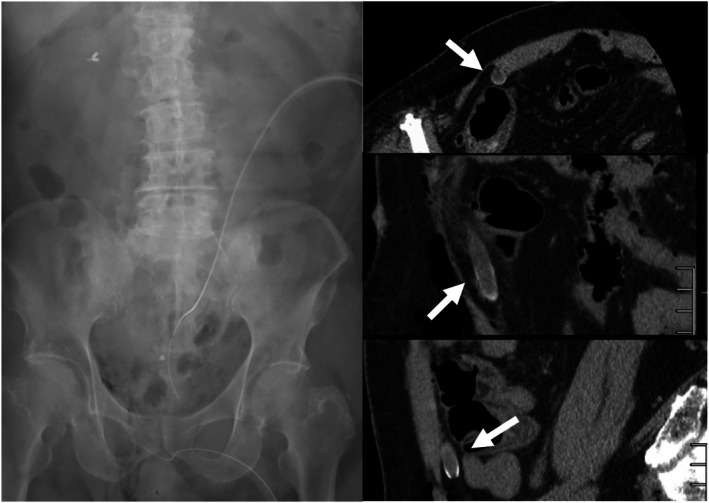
Postoperative imaging for detection of the retained foreign body (tip cover). An immediate postoperative abdominal radiograph obtained in the operating room failed to identify the foreign body, whereas computed tomography (axial, coronal, and sagittal views) successfully detected and localized it. The retained tip cover was located posterior to the right rectus abdominis muscle, above the ventral peritoneal fat, extending in a craniocaudal direction.
